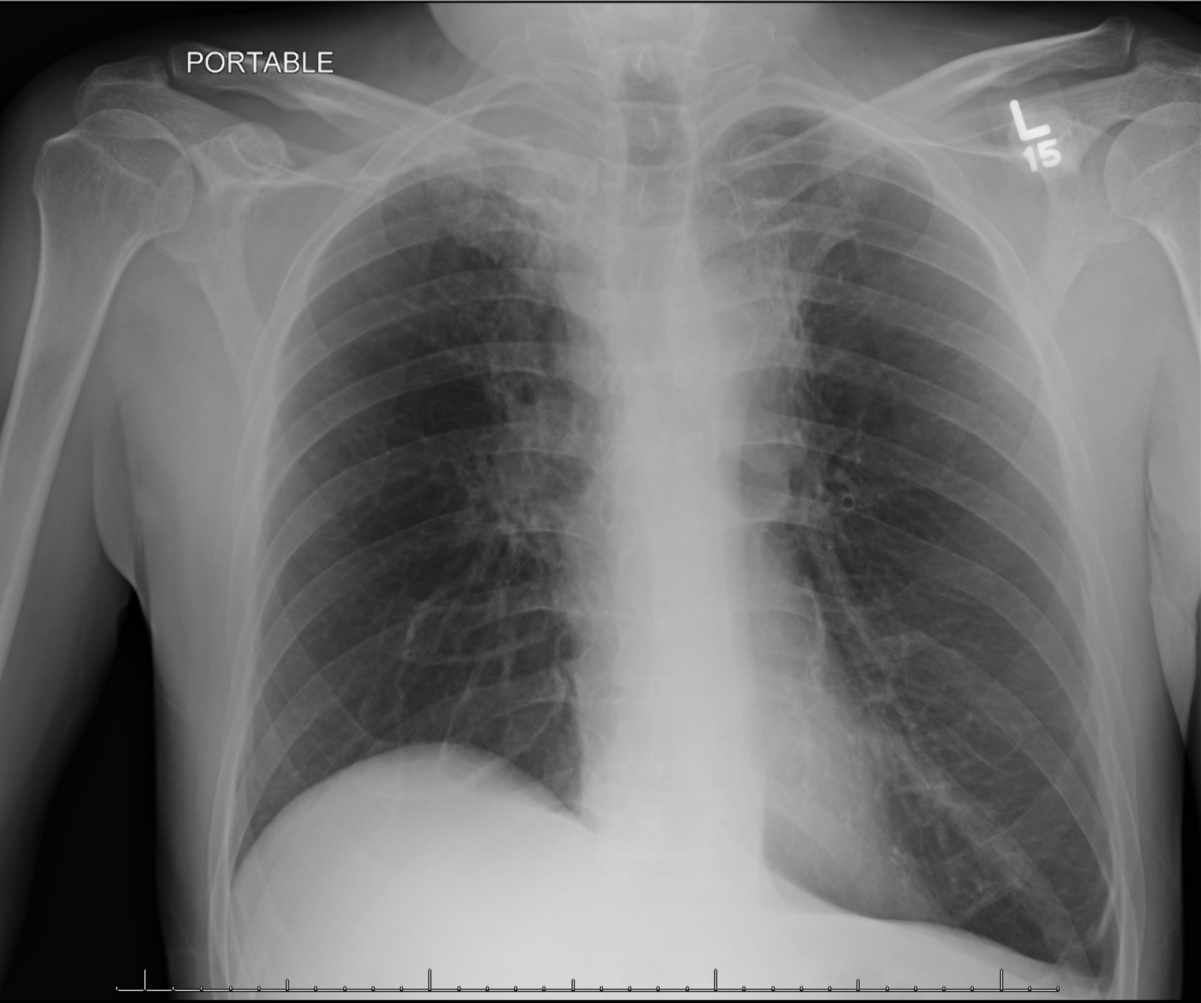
Post-radiation treatment chest x-ray showing fibrotic changes in the lung parenchyma. Chest radiography features suprahilar opacities more on the left, silhouetting the aortic arch and apical pleural thickening right more than left, that represent fibrotic apical changes due to surgery and radiation therapy. There is flattening of the left hemidiaphragm and elevation of the right. There is also pericardiac opacity in the right upper zone of the right lung.
